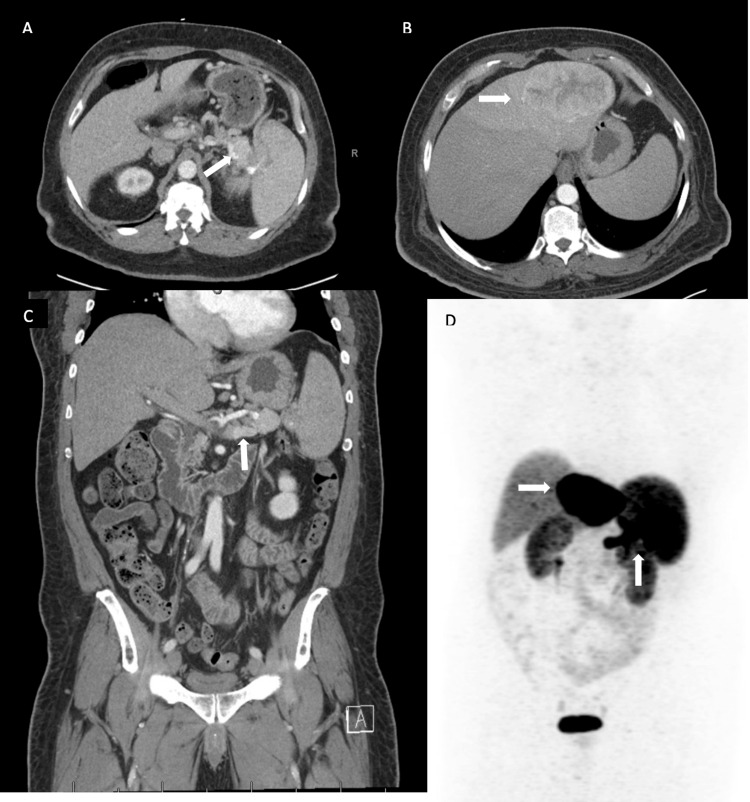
Imaging findings (A) Late arterial phase CT demonstrating pancreatic tail tumor (white arrow); (B) Early arterial phase CT showing liver segment 2 and 3 tumor (white arrow); (C) Late arterial phase CT showing possible splenic vein tumor thrombus (white arrow); (D) Cu-64 DOTATAE PET/CT depicting avidity of the splenic mass, extension into the spleen, and liver metastasis (white arrows).
